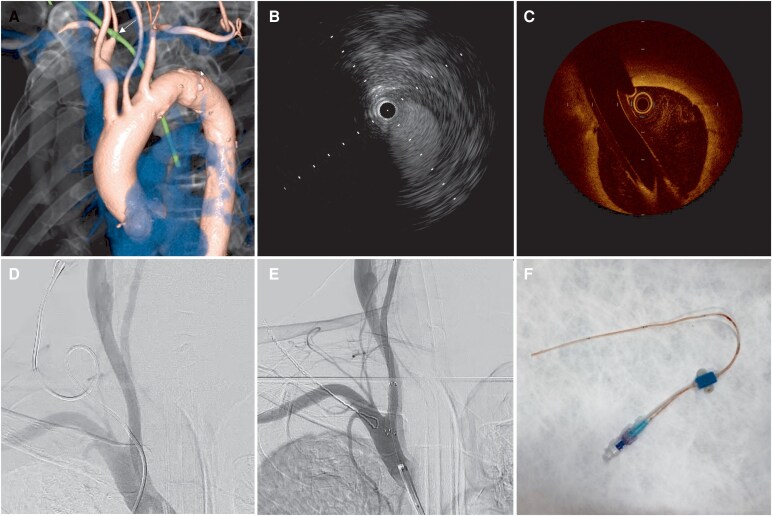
Endovascular therapy for iatrogenic vertebral artery injury using multimodality intravascular imaging. (*A*) Computed tomography revealed the possibility that the central venous catheter had penetrated the right vertebral artery. (*B*) Intravascular ultrasound showed an unusual attenuation in the vertebral artery. (*C*, and Supplementary material online, *Video S1*) OCT clearly demonstrated a tubular structure penetrating the vertebral artery. (*D–F*, and Supplementary material online, *Video S2*) The VIABAHN stent graft was successfully implanted at vertebral artery after removal of the central venous catheter.
